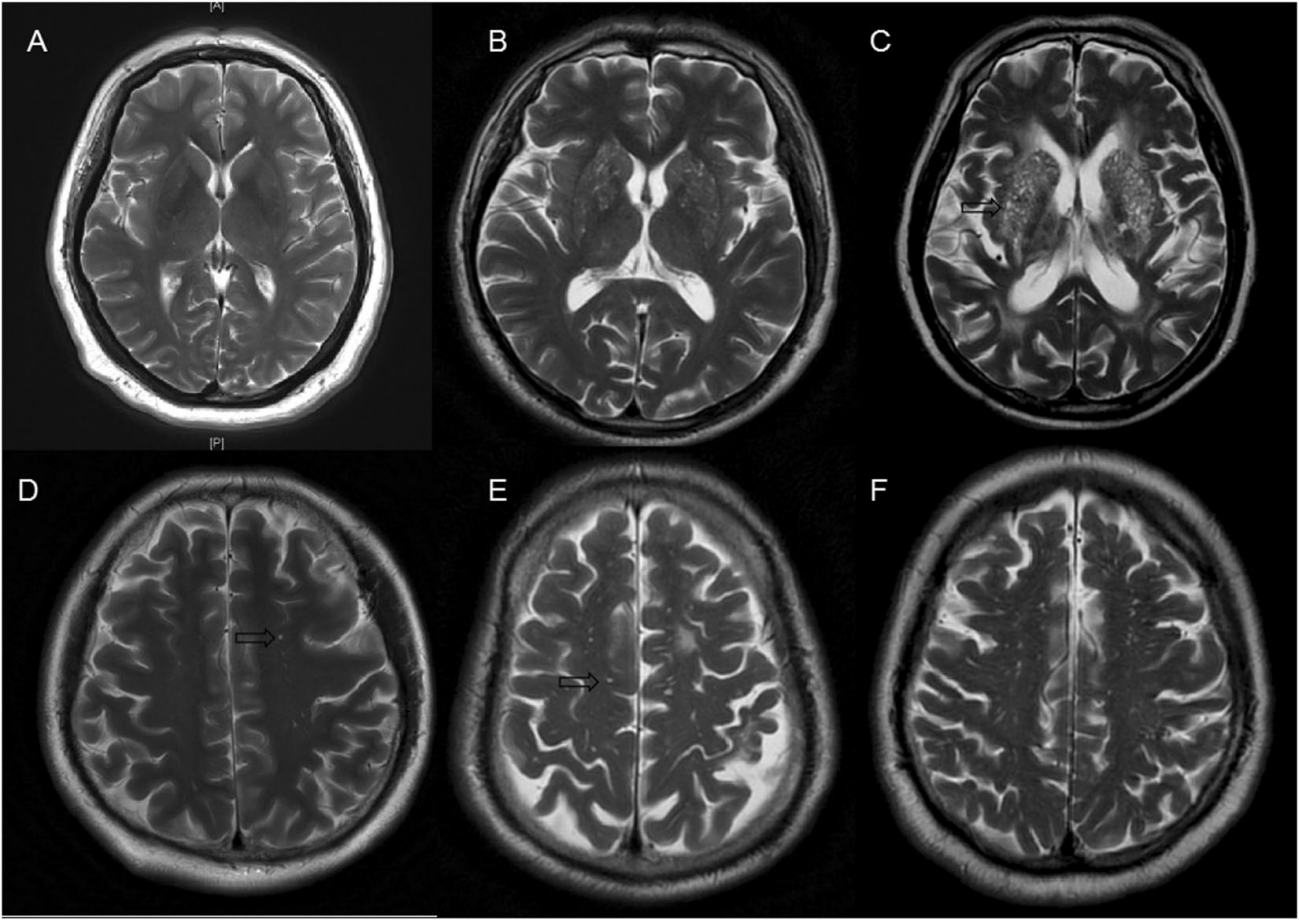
The severity of enlarged perivascular spaces in basal ganglia and white matter. (A) Mild in basal ganglia; (B) moderate in basal ganglia; (C), severe in basal ganglia; (D), mild in white matter; (E), moderate in white matter; (F), severe in white matter. The arrows were pointing to enlarged perivascular spaces, which appear as punctate or linear identical signal intensities that are similar to cerebrospinal fluid on MRI sequences.
